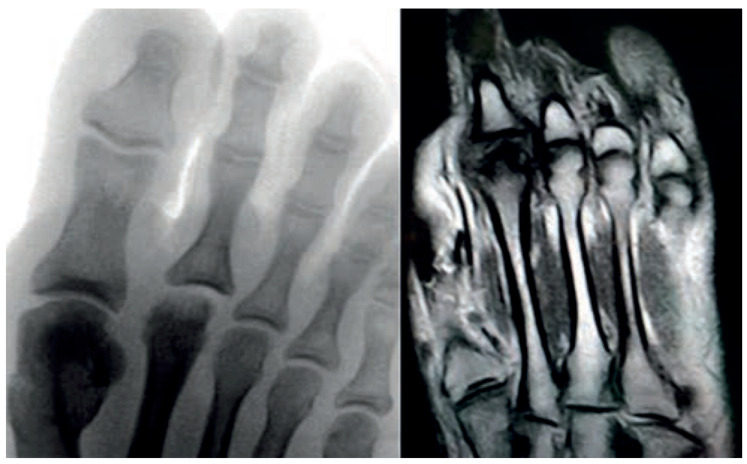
Radiograph (left) and MRI (right) of a 17-year-old female patient showing necrotic changes and initial flattening of the second metatarsal head.
